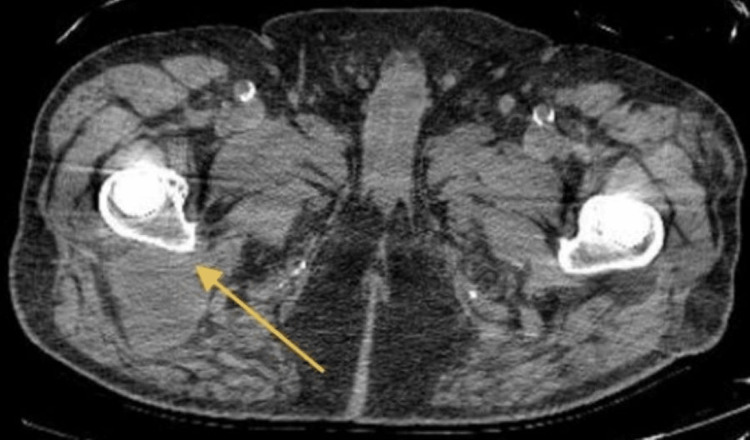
CT pelvis Fluid is present in lesser trochanteric bursa bilaterally. Large 5.2 x 5 centimeter fluid build-up in the right lesser trochanteric bursa.
